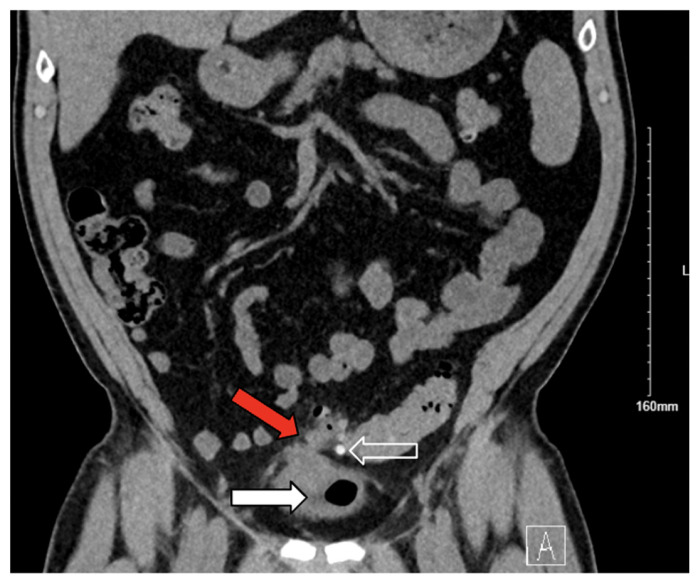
Coronal noncontrast computed tomography showing air within the bladder (white arrow) and superior sigmoid colon diverticulitis (white outlined arrow). A suspected fistulous connection between the sigmoid colon and bladder is indicated (red arrow), consistent with colovesical fistula.
